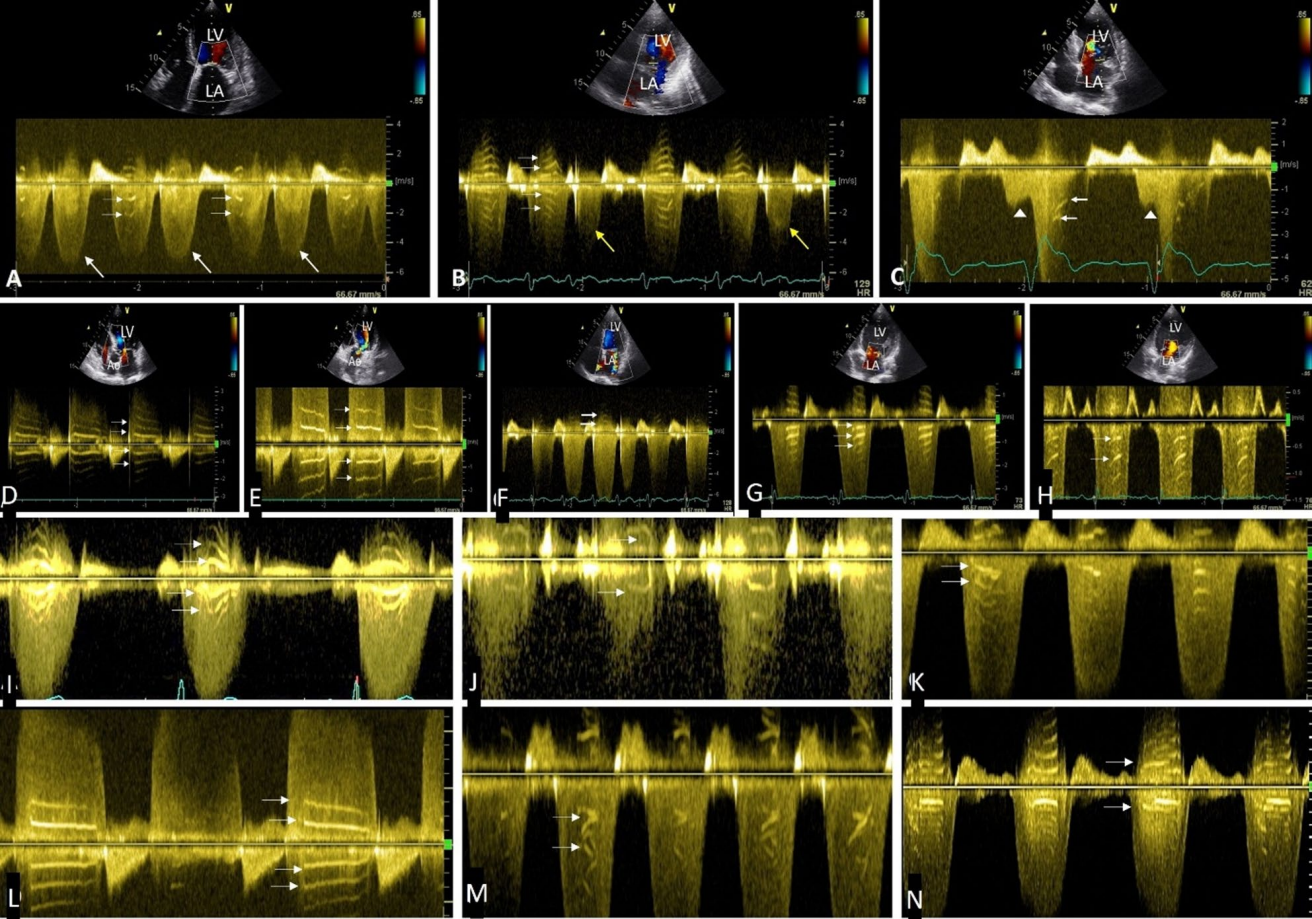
Doppler spectra showing tiger stripes in different situations. (A), stripes are seen in sinus beats (horizontal arrows) but absent in premature beats (white arrows) in a patient with bigeminal rhythm and mitral regurgitation (MR), (B), MR spectrum in smaller cycles during atrial fibrillation (yellow arrows) do not show stripes but stripes (horizontal arrows) are seen in longer cycles. (C). CW Doppler in diastolic MR (arrow heads) in a patent with first degree heart block do not have stripes, whereas stripes (horizontal arrows) are present in systolic MR spectrum. (D). Tiger stripes stand out prominently in a mild aortic regurgitation CW Doppler (arrows) with a relative faint spectrum. (E). Stripes are seen on either side of baseline like mirror image in a PW Doppler spectrum of aortic regurgitation obtained from apical 5 chamber view with sample volume just below the aortic valve. (F). In a relatively denser spectrum the stripes are only seen on opposite side of baseline (arrow) in CW Doppler spectrum of mitral regurgitation obtained from apical 4 chamber view. (G). Stripes in CW Doppler in a faint mitral regurgitation spectrum (Arrows), (H). PW Doppler in the same patient also shows the stripes (arrows). I-N, tiger stripes occurring in different shapes (arrows) in regurgitant Doppler spectra, in curved lines (I), short lines (J, K), horizontal lines (L), oblique lines (M), overlapping lines (N). LV-Left ventricle, LA-Left atrium, Ao-Aorta
